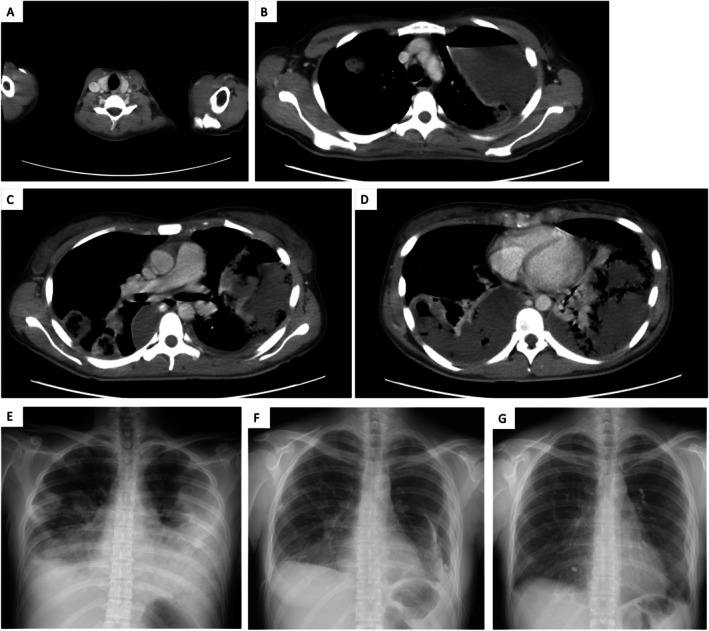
Radiological findings of Lemierre's syndrome and disease progression. (A) Contrast‐enhanced CT on admission showing a thrombus in the left internal jugular vein. (B–D) Axial CT images on admission showing bilateral pleural effusions, with the left effusion encapsulated and containing an air‐fluid level. Numerous cavitary nodules and masses are also visible in both lungs. (E) Chest X‐ray on admission showing bilateral pleural effusions, multiple masses and nodules in both lungs. (F) Chest X‐ray on hospital day 22, prior to EWS placement. Compared with the one on admission, lung suppuration and pyothorax had improved, but new left‐sided pneumothorax was evident. (G) Chest X‐ray on day 106 showing resolution of pneumothorax after EWS treatment.
